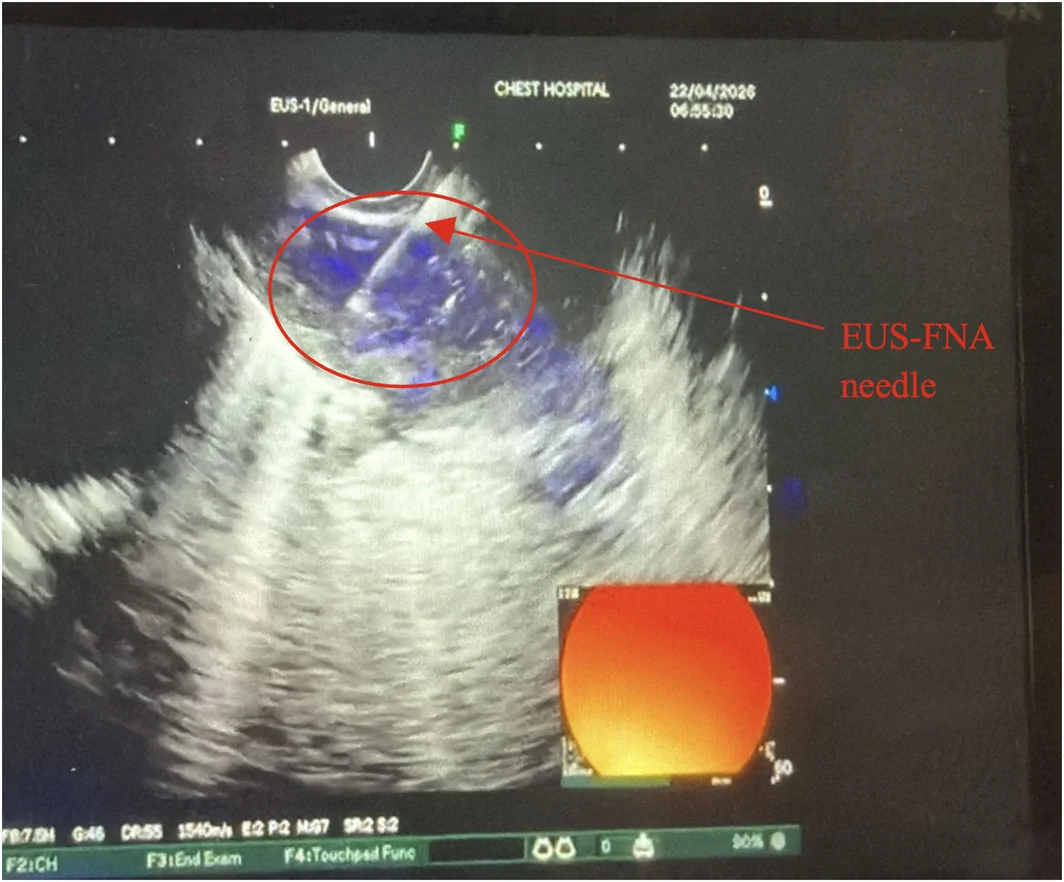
Endoscopic ultrasound‐guided fine‐needle aspiration (EUS‐FNA) of a heterogeneous paraoesophageal left upper lobe lung mass.
